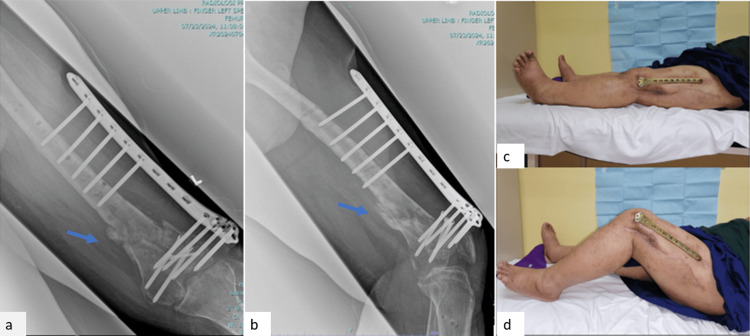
Radiographs and clinical photos at the sixth-month follow-up showing abundant callus formation and a good knee range of motion. (a) Femur radiograph anteroposterior view. (b) Femur radiograph lateral view. (c) Knee in full extension. (d) Knee in full flexion. The blue arrows show callus formation at the fracture site.
